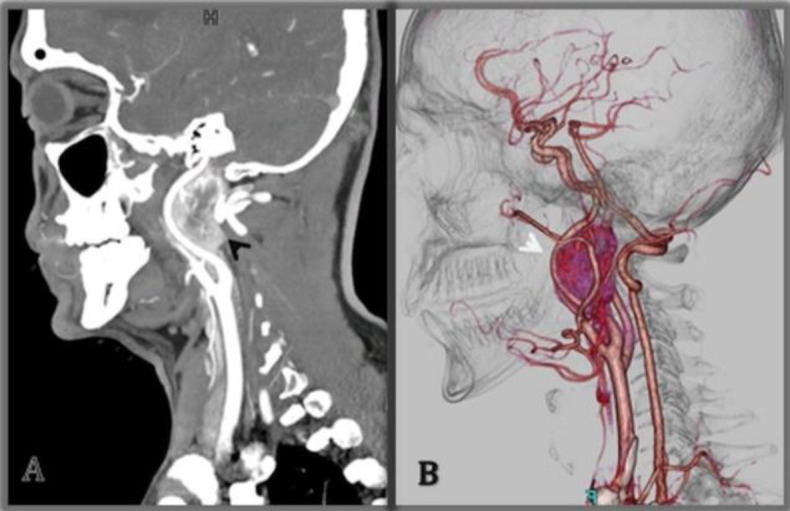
Pre-treatment CT Angiography of Carotid. (A) Post-contrast coronal section and (B) 3D reformatted image. An avidly enhancing highly vascular lesion (arrow head) behind the left internal carotid artery extending form the carotid bifurcation
